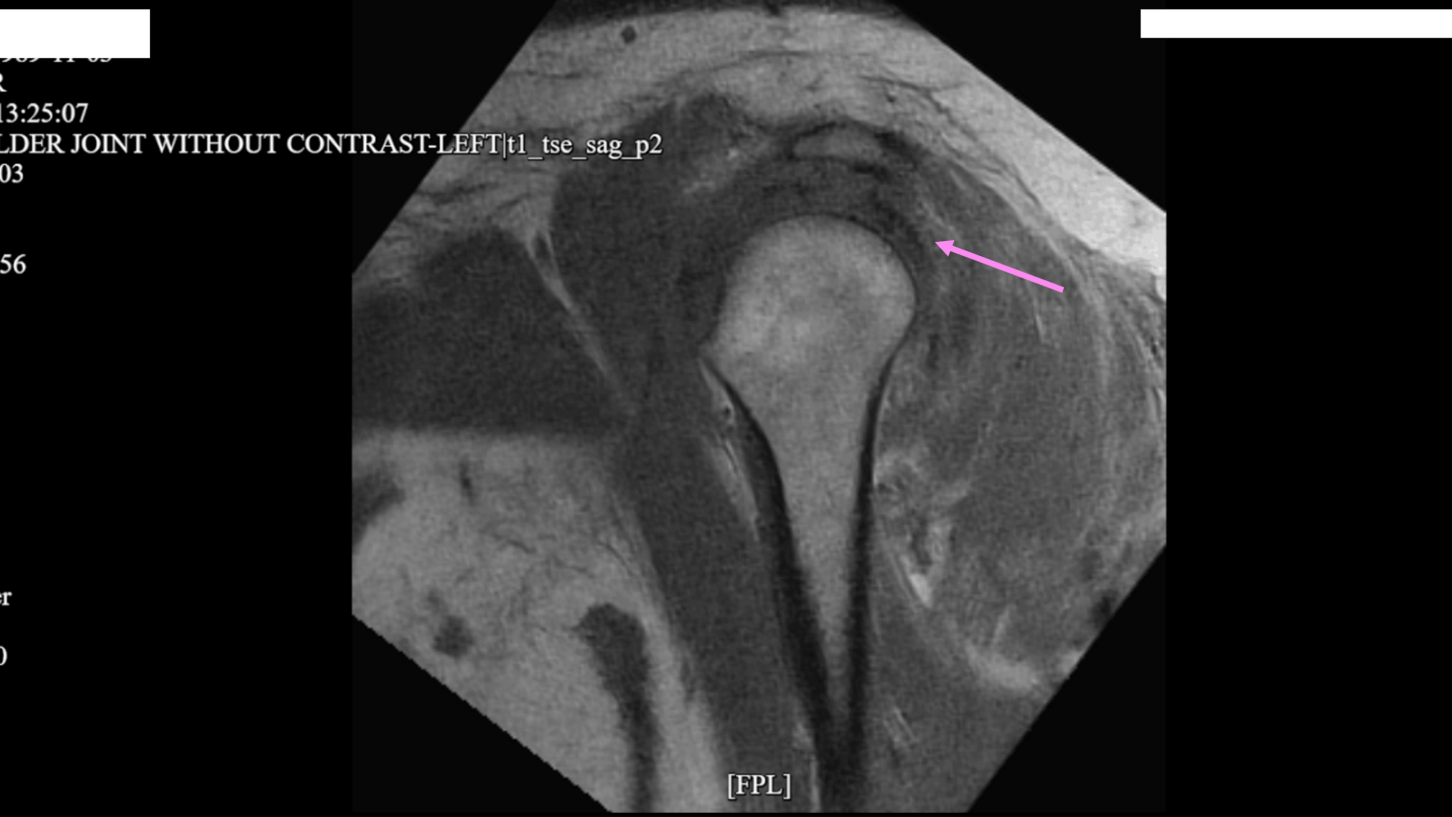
Magnetic resonance imaging without contrast showing small glenohumeral joint effusion on the left upper extremity.
